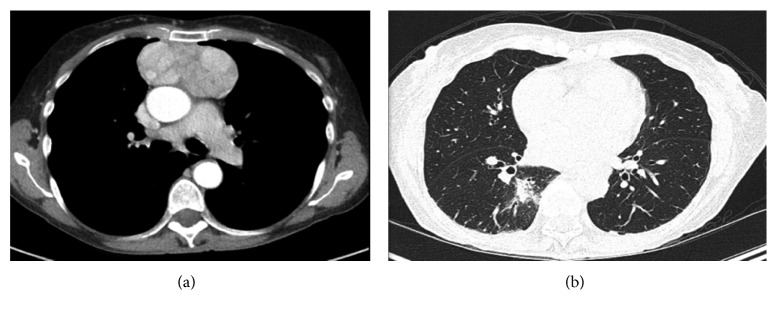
Chest CT: a 4.1 × 8.3 × 7.9 cm mass in the anterior mediastinum with lobulations and dense enhancement (a). Multiple indeterminate nodules throughout the bilateral lungs (b).
